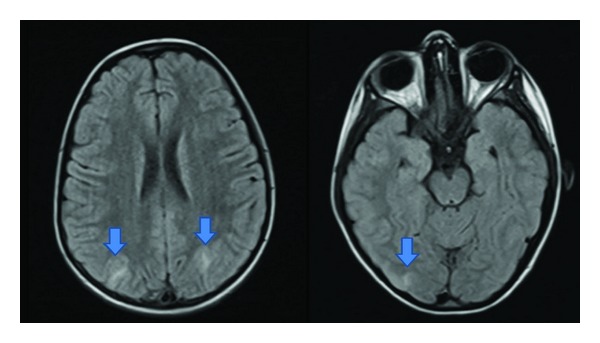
MRI Axial T2-Flair image. A six-years girl with HSP complicated by PRESS. Bilateral hyperintense lesions of subcortical white matter in the occipital region.
